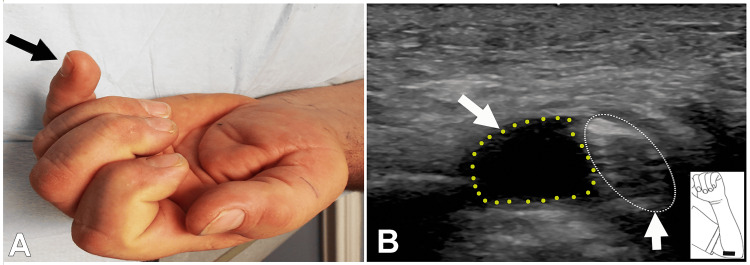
"Nail File Sign" and a Cyst at the Elbow Compressing the Ulnar Nerve Detected on an Ultrasound Study Case 1: (A) "Nail file sign" of the left hand: inability to flex the small finger at the distal interphalangeal joint (arrow). (B) Ultrasound study demonstrating a cyst at the elbow compressing the ulnar nerve (vertical arrows point to the ulnar nerve; oblique arrow points to the cyst).
